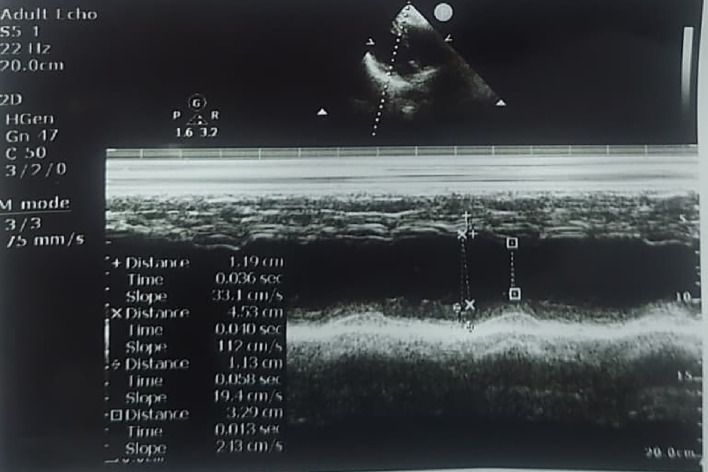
2D Echocardiogram of Male Breast Cancer Patient. The 2D Echocardiogram showed normal cardiac structure with normal ejection fraction
